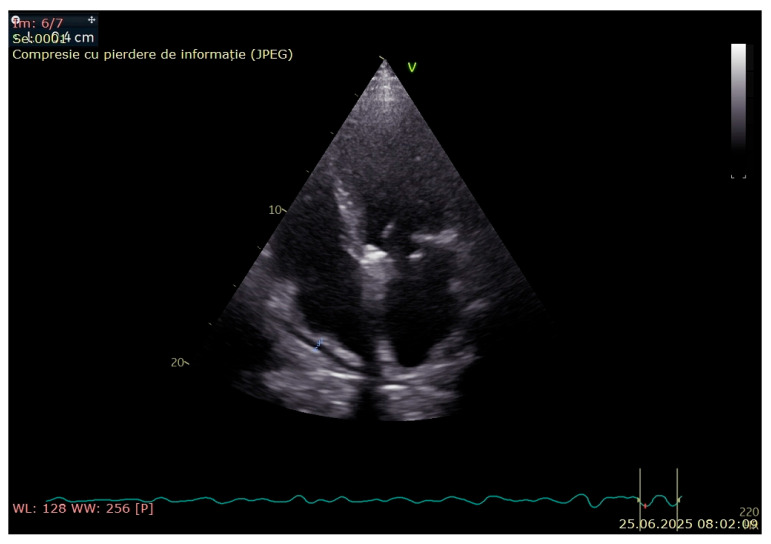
The 1-month follow-up echocardiography.
